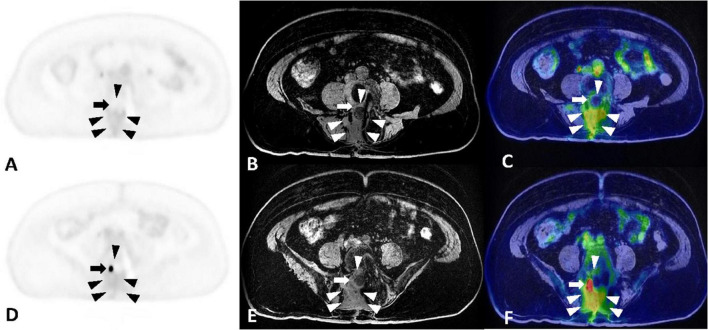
(A–C) Maximum intensity projection, MRI and PET-MR fusion images at the level of L3-L4, separately. (D–F) Maximum intensity projection, MRI and PET-MR fusion images at the level of L5-S1, separately. Fat-suppression T1-weighted sequences in the axial plane without contrast showing increased and homogeneous intensity within the epidural space [arrowheads in panels (B,E)], indicative of extensive scar formation. The fusion images [arrowheads in panels (C,F)] reveal mild FDG uptake in the epidural scar with SUVmax of 2.0. The epidural fibrosis encircles the right L4 root [arrow in panel (C)] and right S1 root [arrow in panel (F)]. The FDG uptake of the right L4 root is similar to that of the adjacent epidural scar, while the right S1 root shows intense FDG uptake with SUVmax of 4.2.
